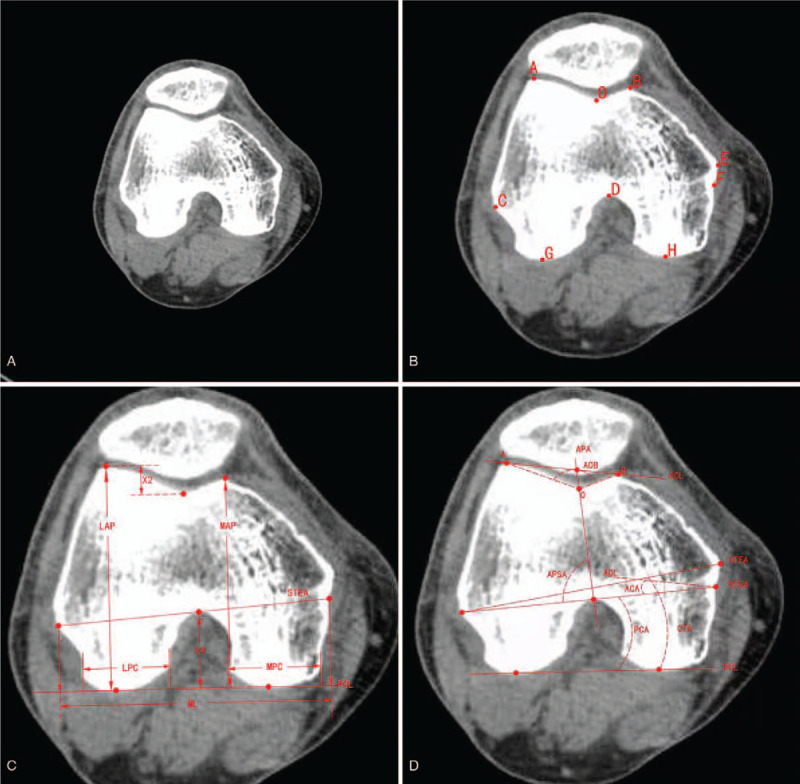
Measurement parameters for the distal femur. A Computed tomography (CT) image of the distal femur. B Location of landmarks on an axial CT image. *A* Highest point of the lateral condyle. *B* Highest point of the medial condyle. *C* Most prominent point of the lateral epicondyle. *D* Top of the intercondylar notch. *E* Most prominent point of the medial epicondyle. *F* Most concave point of the medial epicondyle. *G* Most prominent point of the lateral posterior condyle. *H* Most prominent point of the medial posterior condyle. *O* Most distal point at the bottom of the trochlear groove. C Morphological parameters of the distal femur. *LAP* vertical distance from the highest point of the external epicondyle of the femur to the PCL, *MAP* vertical distance from the highest point of the medial epicondyle of the femur to the PCL, *LPC* the lateral posterior condyle width, *MPC* the medioposterior condyle width, *X2* the depth of the anterior patellar groove, *X4* the intercondylar notch, *ML* the most concave point of the distal medial femoral condyle and the most prominent point of the external condyle, the distance after the vertical projection of the connection line at the PCL. D Rotational axis and angle of the distal femur. *ACA* angle between ACL and STEA, *PCA* angle between PCL and STEA, *APSA* angle between APA and STEA, *CTA* angle between PCL and CTEA.
